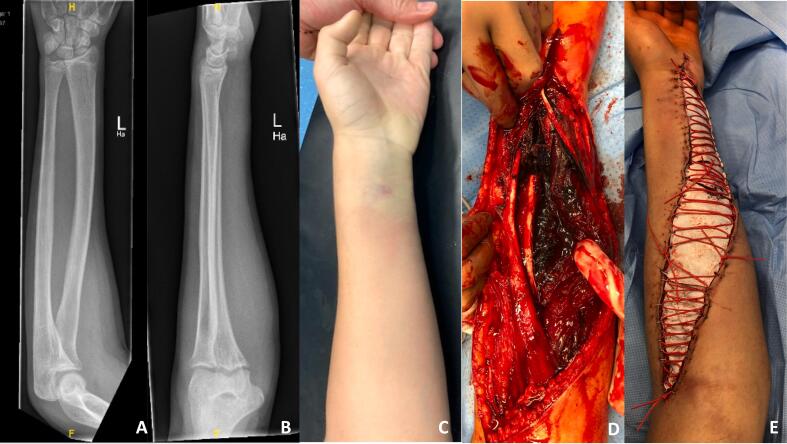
Conventional X-ray in two planes of the injured forearm of the 14-year-old boy showed no sign of fracture (A+B). Upon presentation to our emergency room, the left forearm and hand displayed pronounced circulatory disturbance, manifested by a pale hand (C). Intraoperatively, a hematoma was identified within the deep flexor tendon compartment following decompression fasciotomy (D). Flexor sided wound defect with “Roman sandal wound closure” with EpiGard® after decompression fasciotomy (E).
